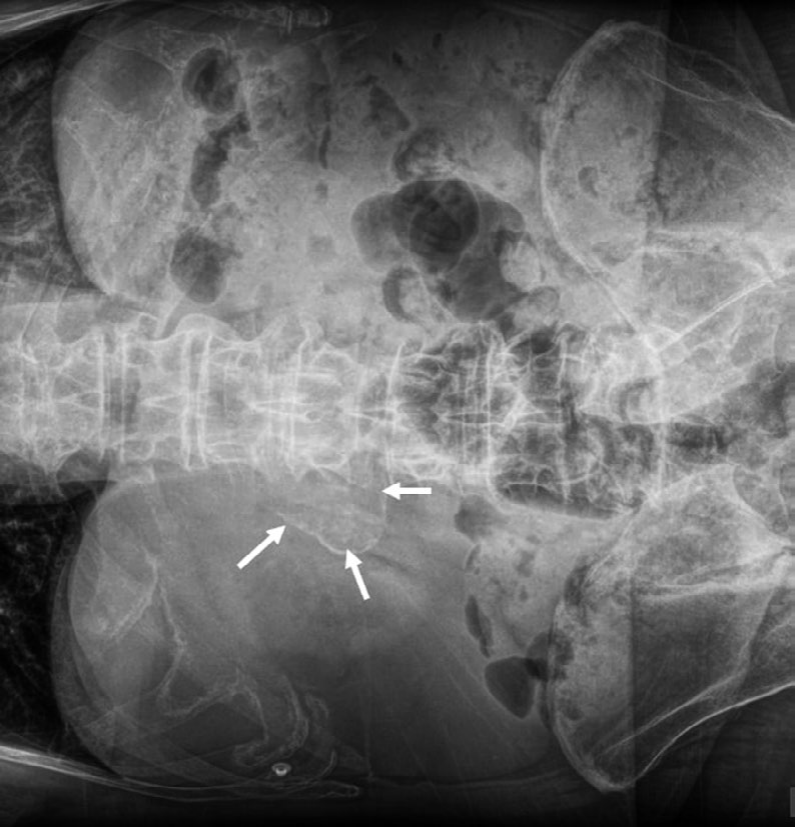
Abdominal radiograph showing an ossified mass projecting at the right paraspinal area (arrows)
